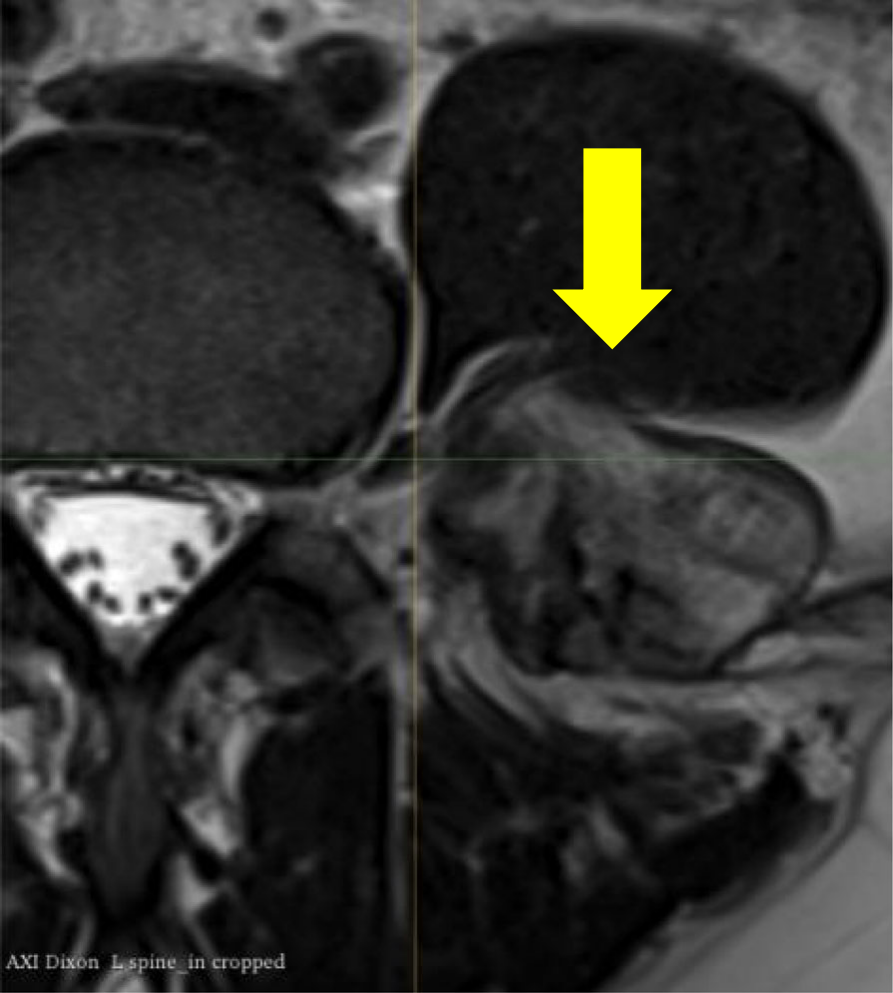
MRI demonstrating the L3 nerve root anterior to the osteochondroma (arrow)
